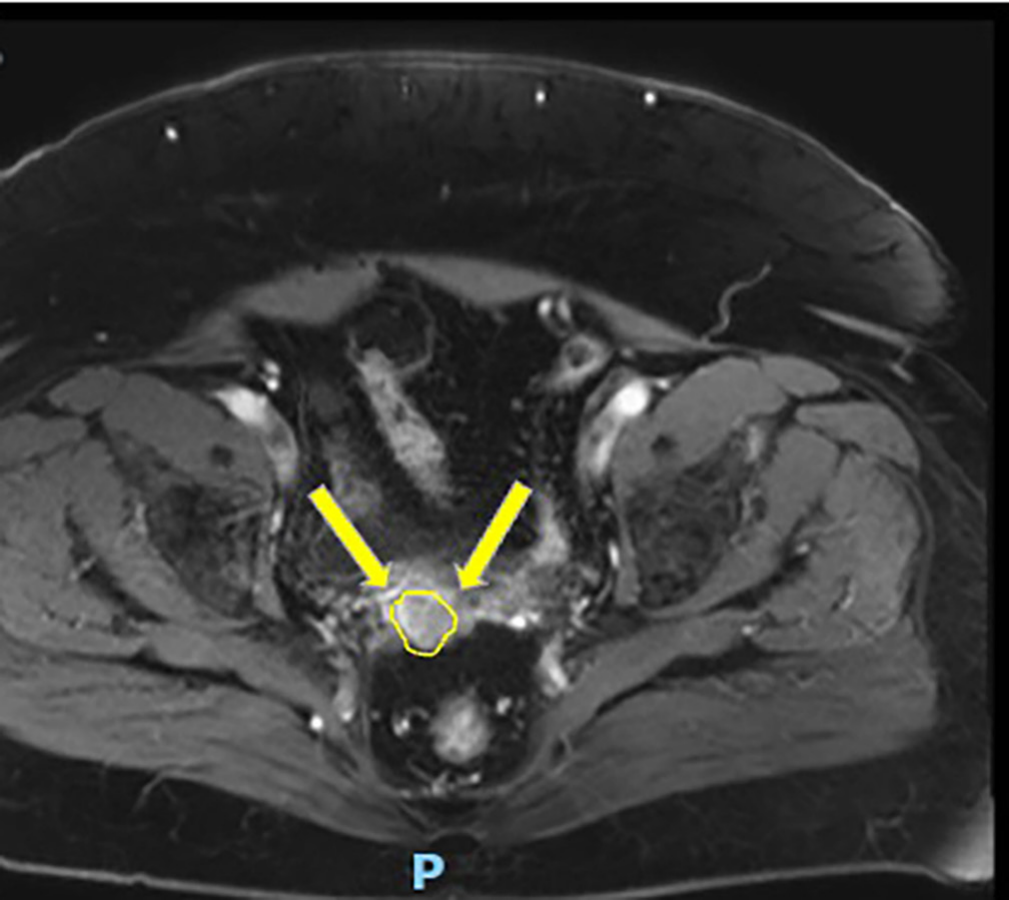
MRI image showing the first recurrence in the vaginal vault. Arrows highlight a nodule of 33×21×21 mm.
